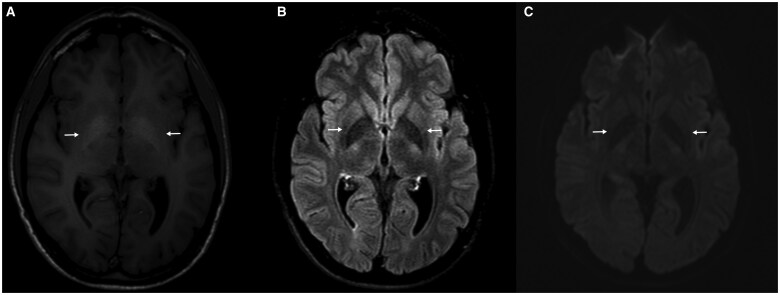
Brain MRI findings. Precontrast T1-weighted images (A) demonstrate a symmetric hyperintense signal increase in both globus pallidus (arrow), without a corresponding signal increase on T2-FLAIR (B) or diffusion-weighted images (C).
